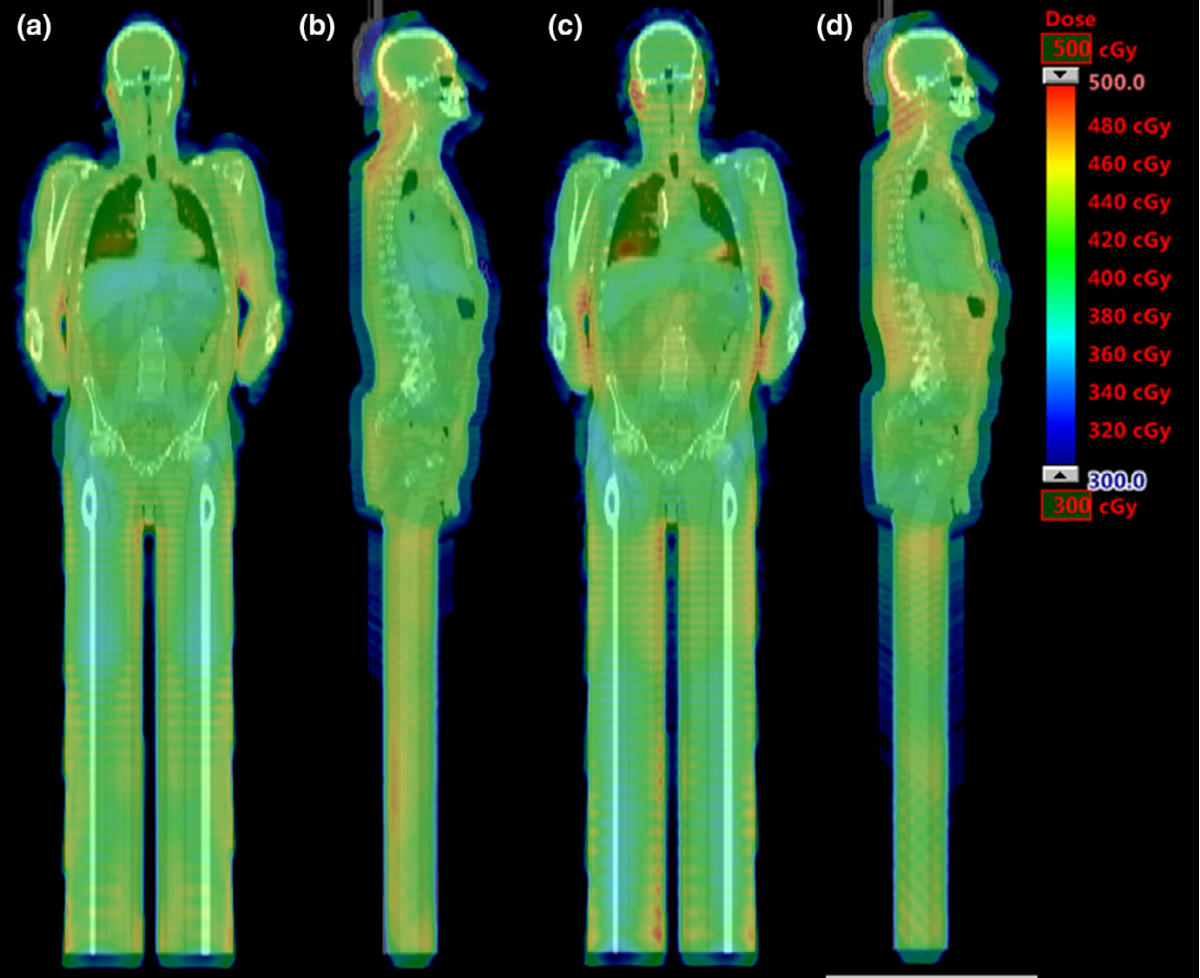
CT data of single coronal and sagittal slices from the same patient in the 21‐23 cm Tall categorization with dose color washes corresponding to each TBI plan. (a) and (b) show the clinical plan dose wash (clinicalTBI), and (c) and (d) the standard FFF plan dose wash (fffTBI).
